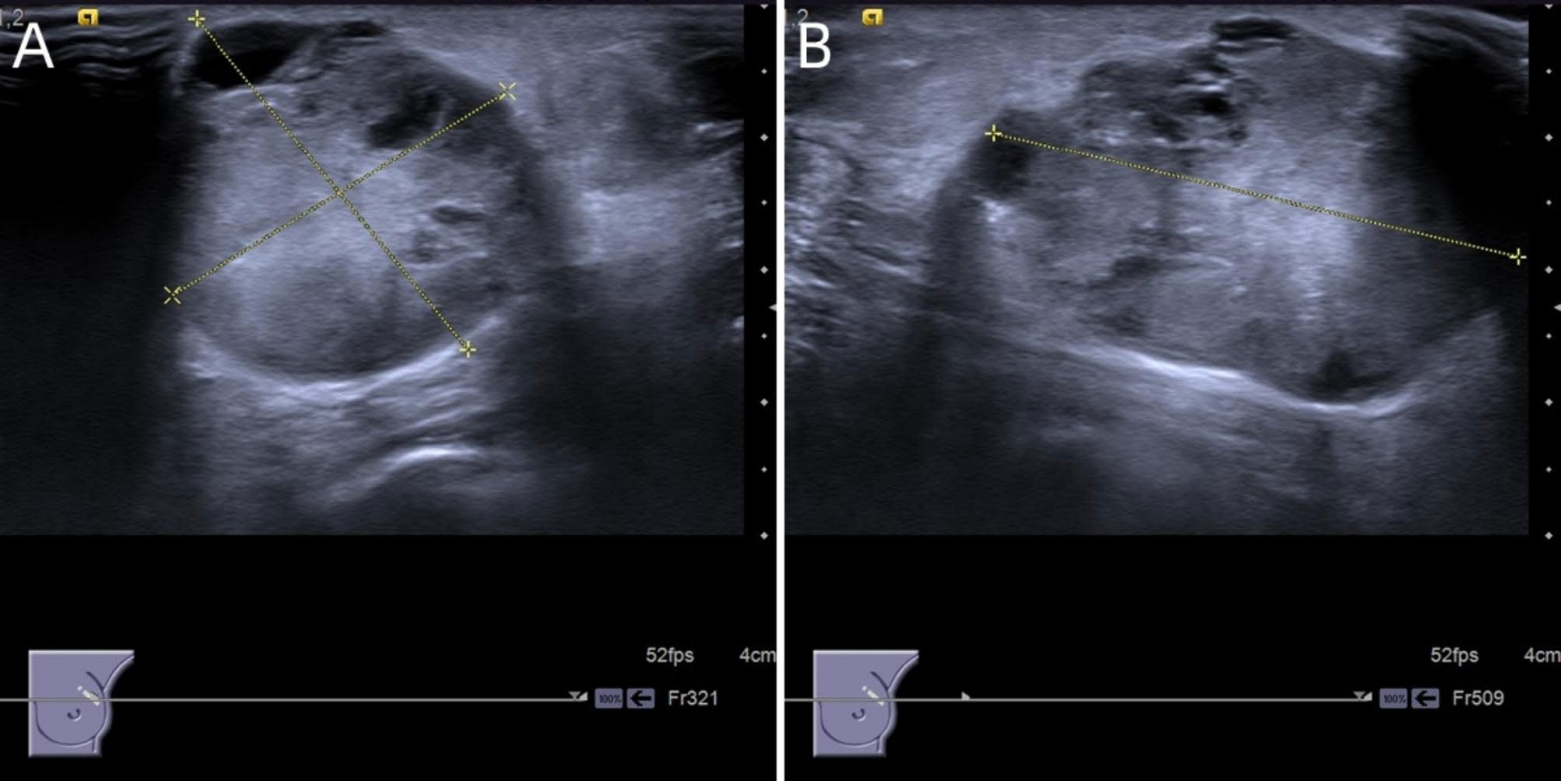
Ultrasound findings of this case. In the left breast, there is an elliptic mixed echoic mass dominated by a solid hypoechoic mass with smooth edges (A, B)
